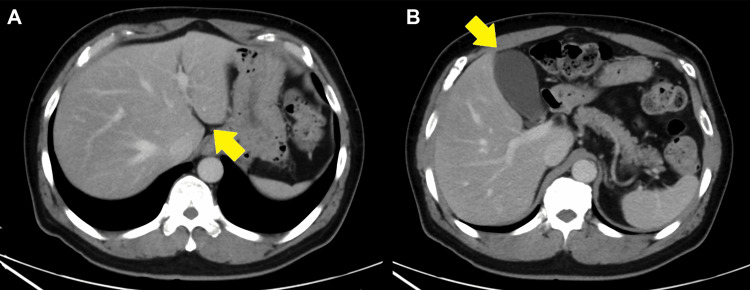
Computed tomography scan with arterial phase contrast on the first day. (A) No abnormalities in the arteries, portal veins, or bloodstreams of other organs were observed, although the portal veins were not clearly enhanced. (B) No gallbladder infarction, thickening of the gallbladder wall, or discontinuation of the gallbladder artery was observed.
